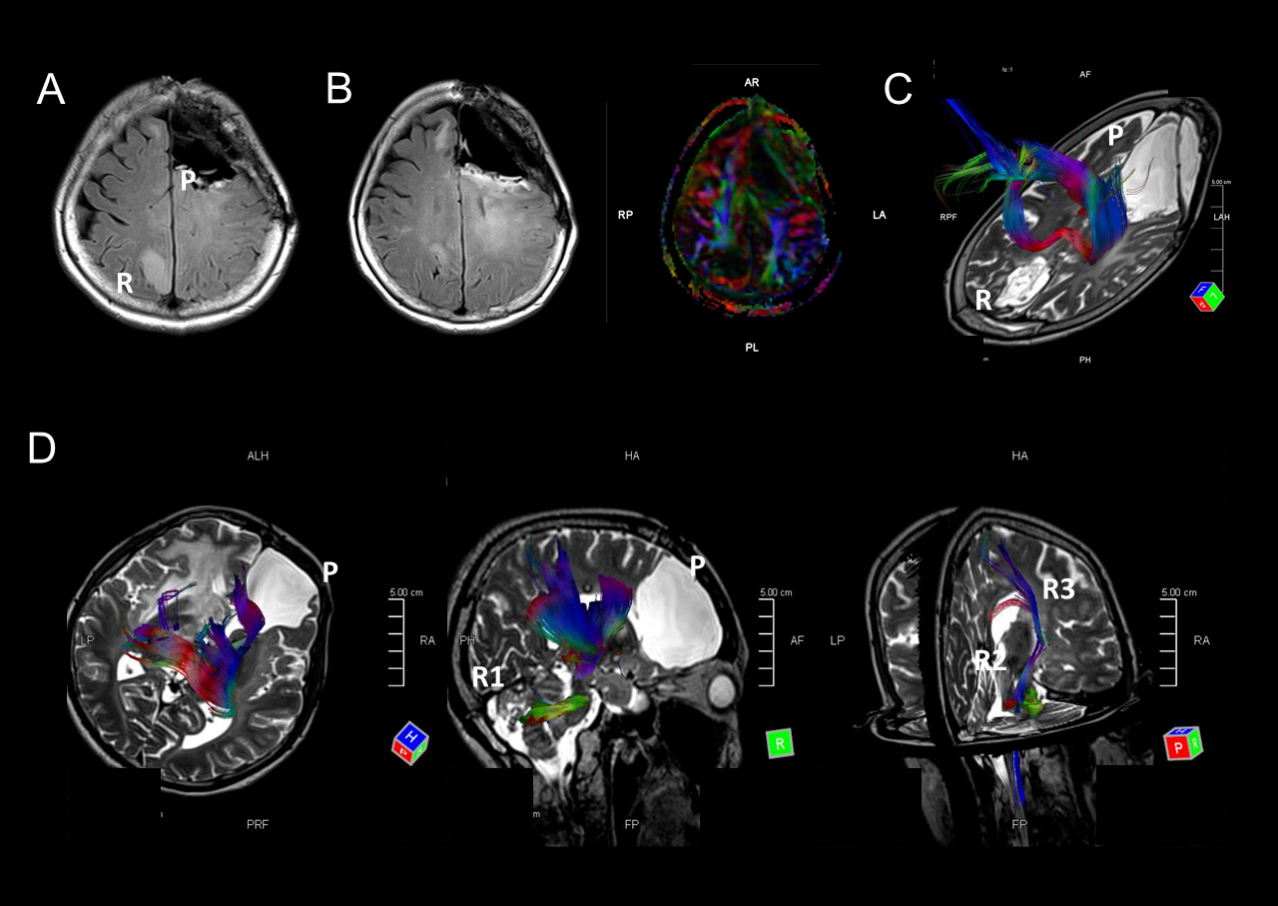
A-C. MRI images of case M1. A FLAIR image indicates that the tumor metastasized from the left frontal lobe to the opposite parietal lobe (A). FLAIR and DTI images at almost the same level indicate that the FLAIR high-intensity areas overlap with a major fiber bundle detected by DTI (B). A fiber tracking image indicates that fiber bundles connect the primary and recurrent tumor areas (C). D. Fiber tracking images show fiber bundles connect the primary tumor area to the right cerebellum *via* the right thalamus and brain stem. Other fiber bundles were detected from the brain stem to right basal nucleus. MRI images of this patient are shown in Figure 1F-1H.
